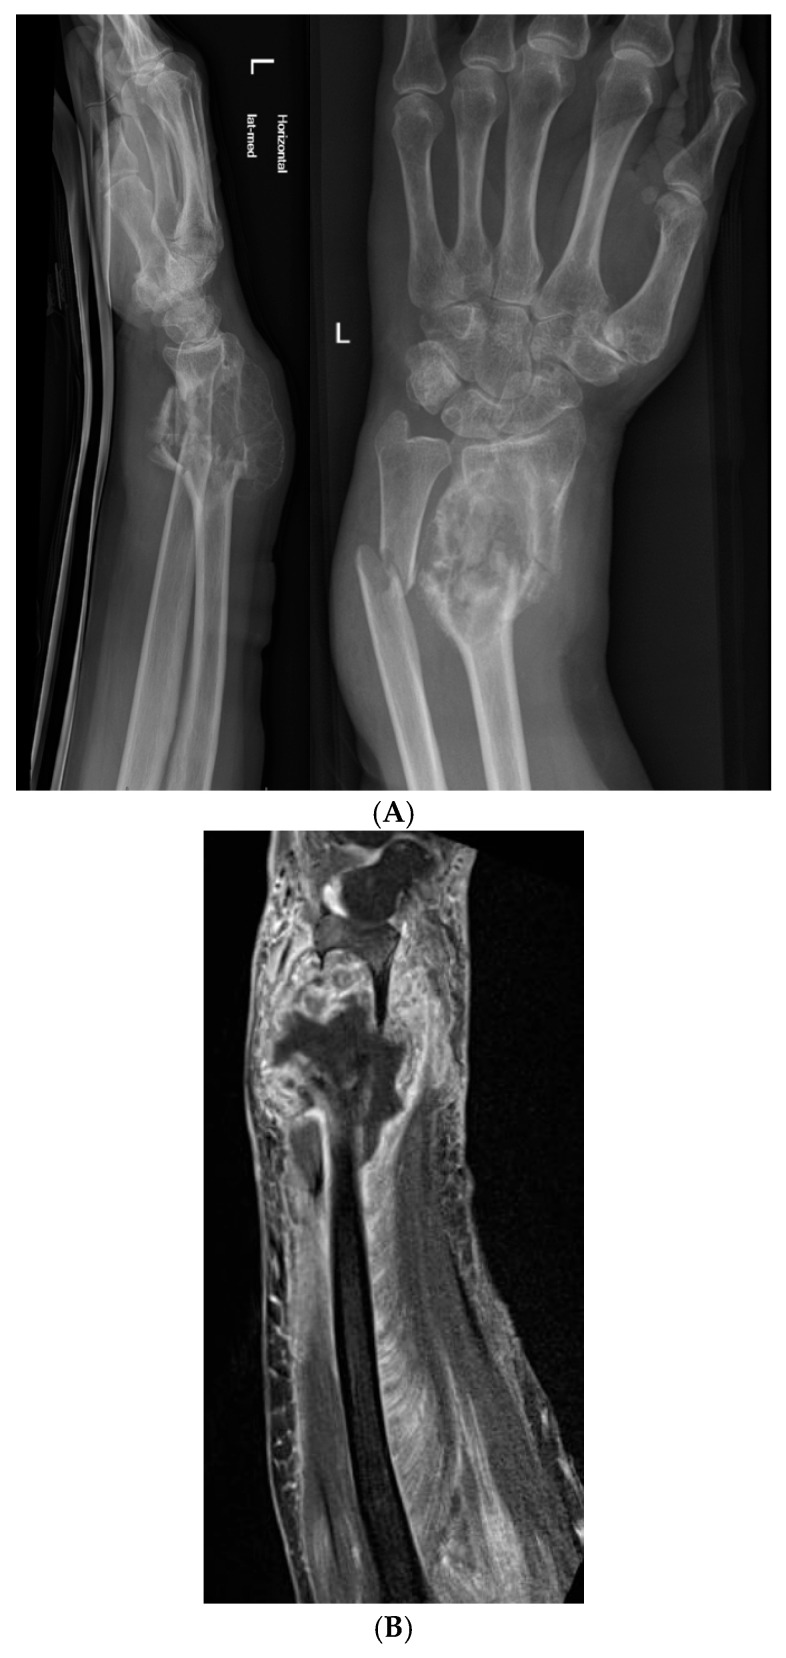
(A) X-ray in two planes of the perilesional fracture, (B) coronal T2 MRI scan.
